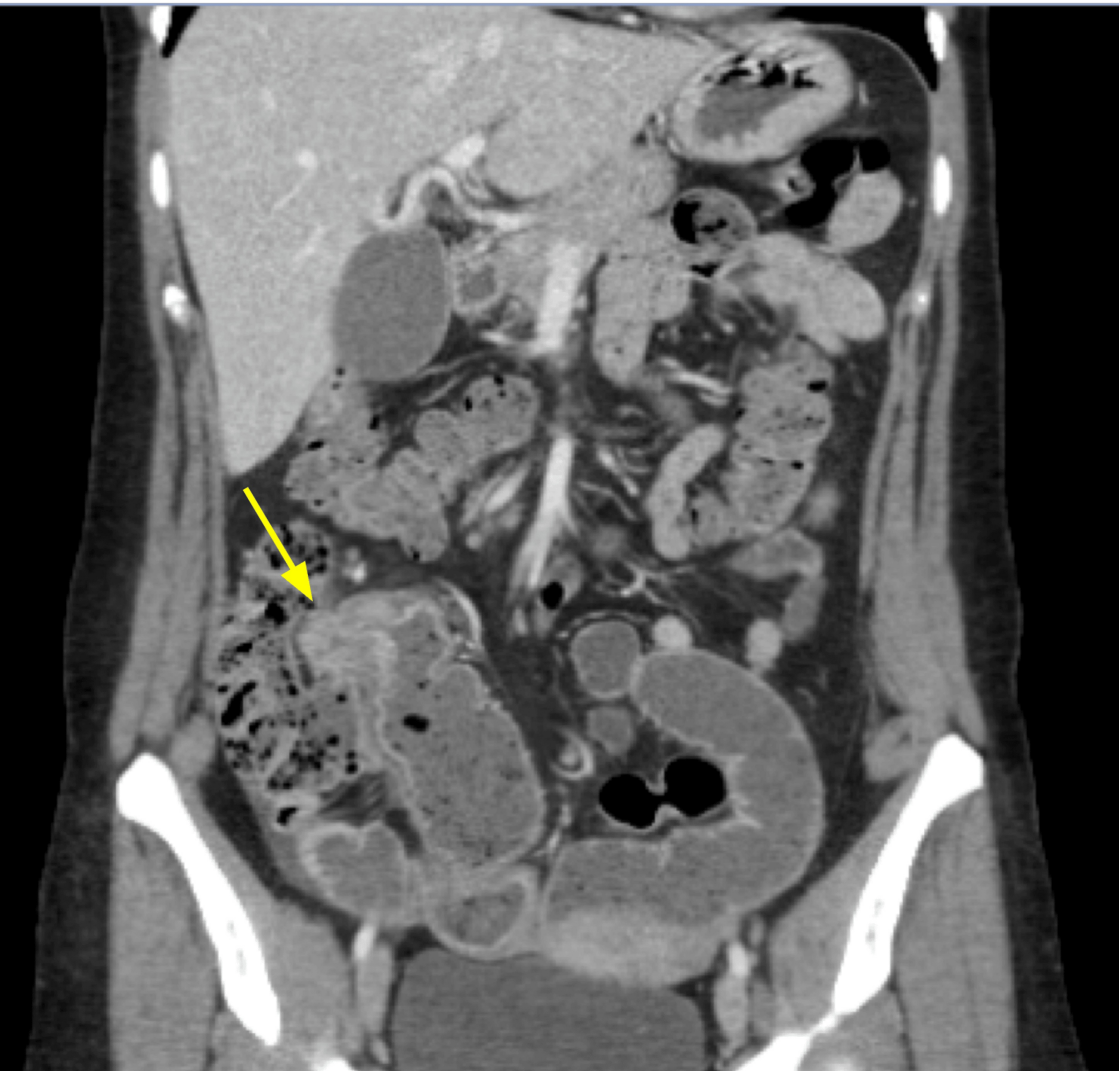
Contrast-enhanced CT scan of the abdomen and pelvis showed marked thickening, inflammation, and stricturing at the terminal ileum (yellow arrow) with multifocal mild to moderate strictures proximally. No fistulas, abscesses, or adenopathy were identified.
